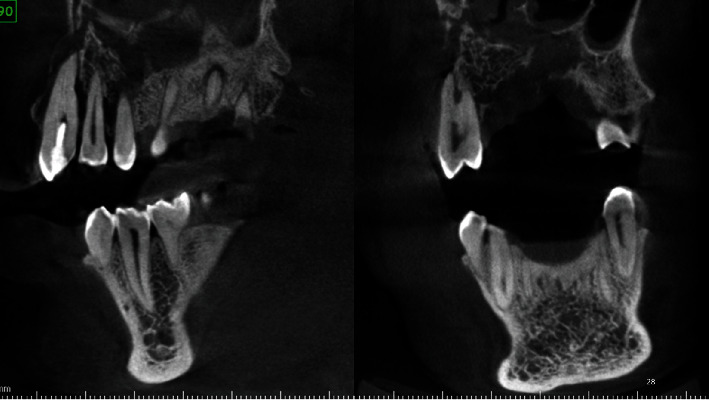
CBCT sagittal and coronal views of case 2 showing bony sequestrum with gross osteolytic changes infiltrating the floor of the maxillary sinus.
